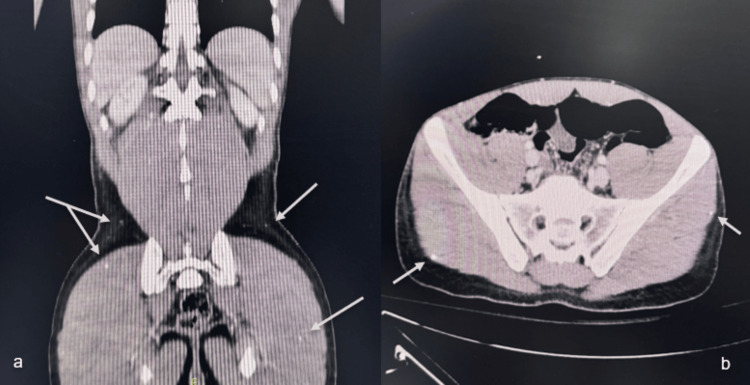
Enhanced computed tomography scan of the lower chest, abdomen, and pelvis revealing multiple muscular and cutaneous foci of calcification as marked by the arrows, in the a) Coronal, b) Axial views.
